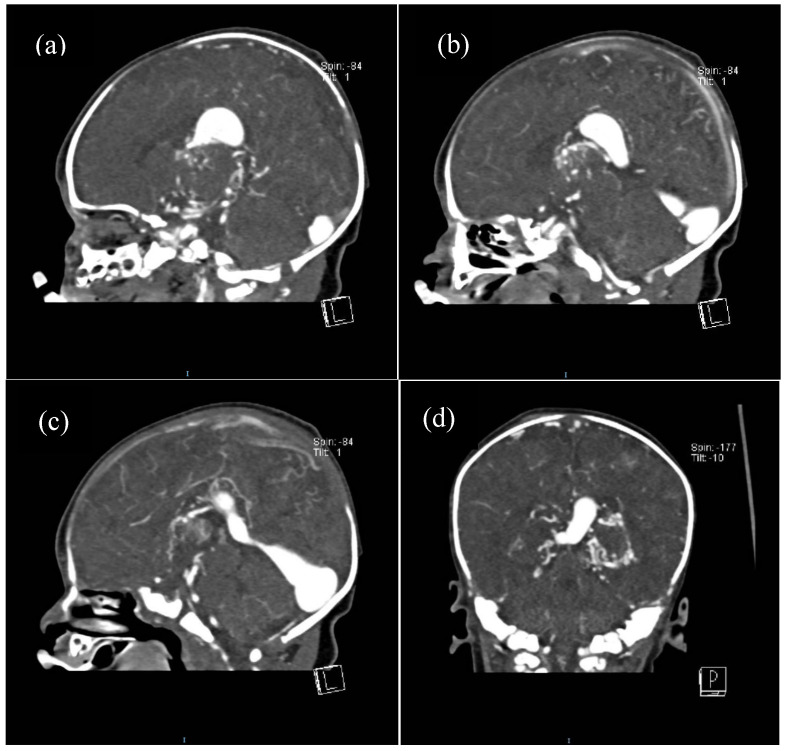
Contrast cerebral CT scan: (a–c). Progression of filling with contrast substance of the VoGM (sagittal view), (d). VoGM filled with contrast in coronal plane (posteroanterior view).
